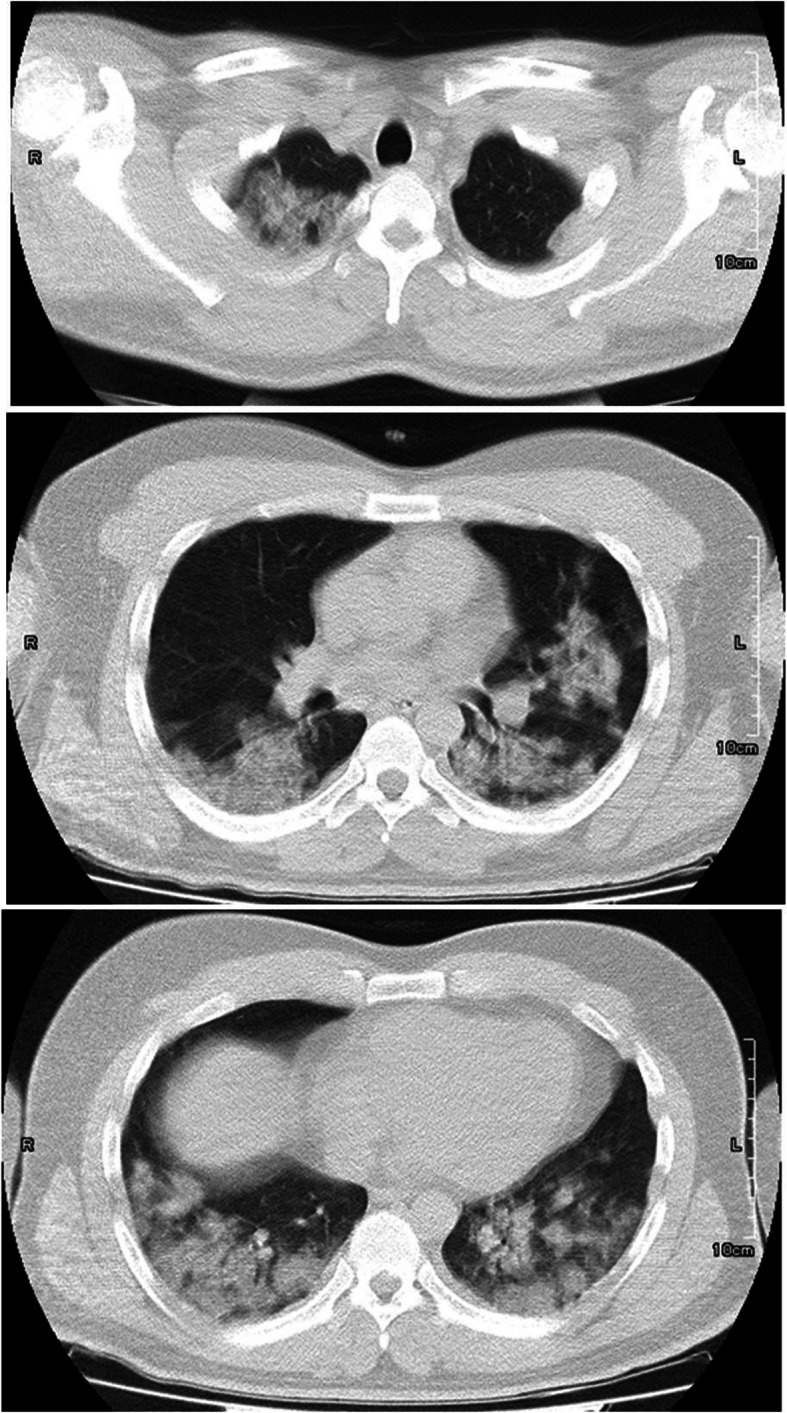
Axial without contrast-enhanced chest computed tomography (CT) image showing a coronavirus disease (COVID-19) infection
